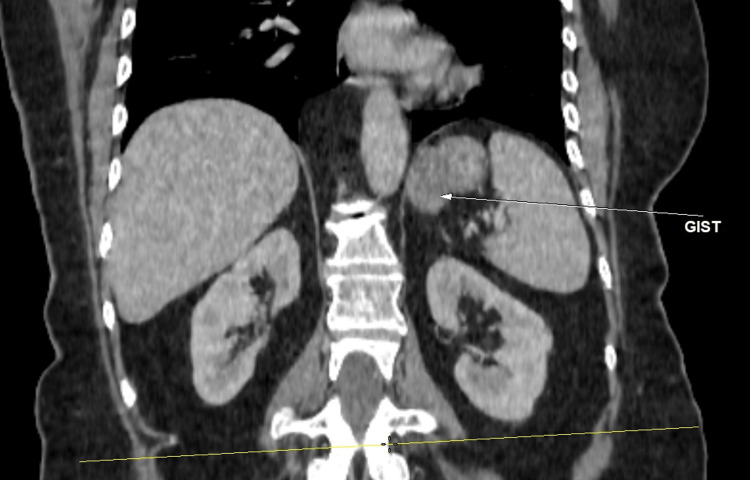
Abdominal CT in the coronal section The image shows a 5 cm solid, exophytic, ovoid mass in the gastric region with no evidence of invasion into adjacent tissues and irregular borders, which is indicative of GIST (arrow).
